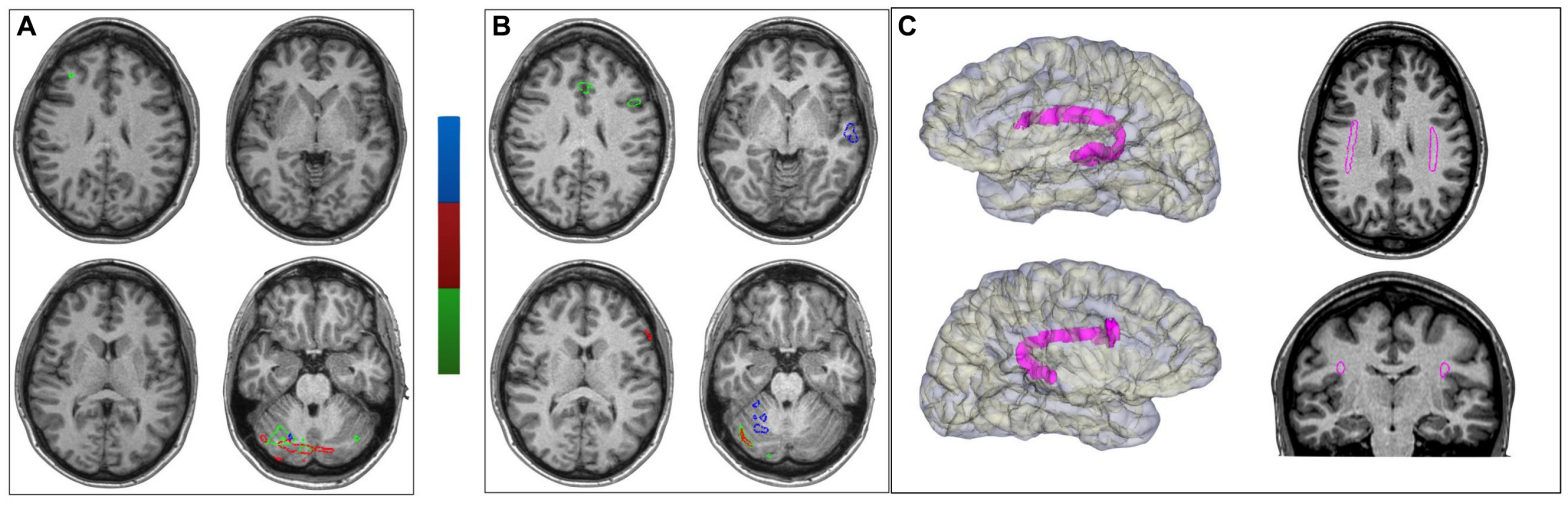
Functional MRI and DTI. (A) Functional MRI with language-dedicated tasks (phonemic and semantic fluency in green, auditory comprehension in blue and naming by verbal description in red). On the left side, the preoperative functional MRI showing a right cerebellar lateralization, with only a slight activation at the level of the right inferior frontal gyrus (the other two tasks were inconclusive). (B) The post-thermocoagulations functional MRI confirming the right cerebellar activation with left-side supratentorial activation either at the level of the inferior frontal gyrus for fluency and posterior temporal regions for comprehension. (C) Bilateral tractography representation of the superior longitudinal and arcuate fasciculi (SLF-III/AF) complex. On the left side: 3D surface rendering of each hemisphere and SLF-III/AF complex (in magenta). On the right side: axial and coronal slice of T13D brain-MR with representation of SLF-III/AF complex (in magenta). Left-side fasciculus volume resulted larger than the right-side one.
